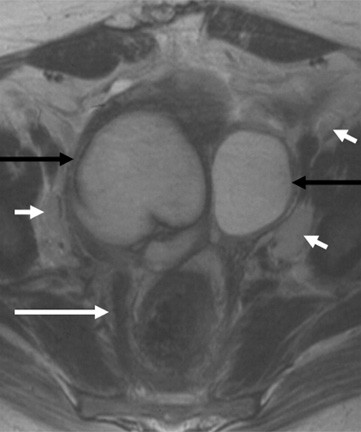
MRI axial T2W images of the pelvis. Bilateral adnexal cystic masses are demonstrated (black arrows). The cyst wall on the right side is thick and irregular. There is marked thickening of the mesorectal fascia (long white arrow). Multiple prominent nodes are demonstrated along the pelvic sidewalls, which are very high in signal intensity on T2W (short white arrows).
